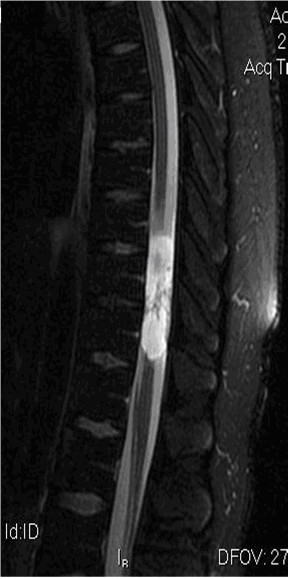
Sagittal T2-weighted thoracic magnetic resonance image. Note intradural extramedullary lesion at T9-T11 (arrow).
